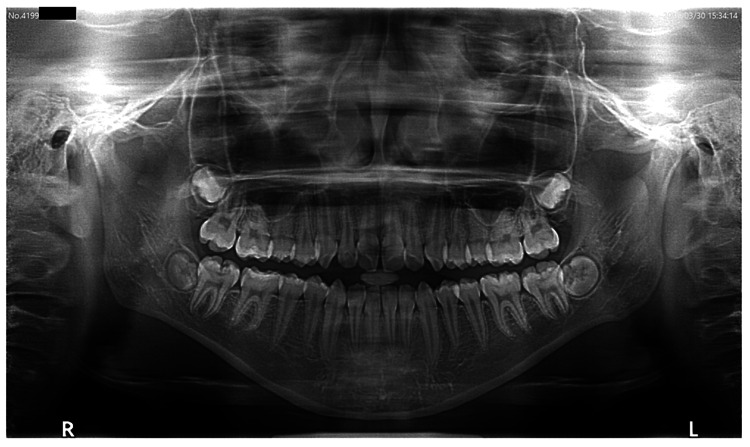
Panoramic radiography at the initiation of treatment in Case 2
